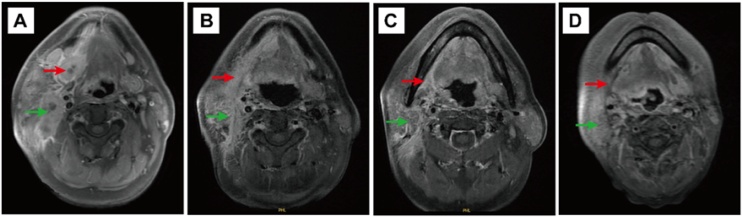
Magnetic Resonance Imaging (MRI) revealing the tumour (red arrow) in the right submandibular gland with suspicious metastatic lymph nodes (green arrow) (A). Follow-up MRI scans at 12-months (B), 24-months (C), and 36-months (D).
